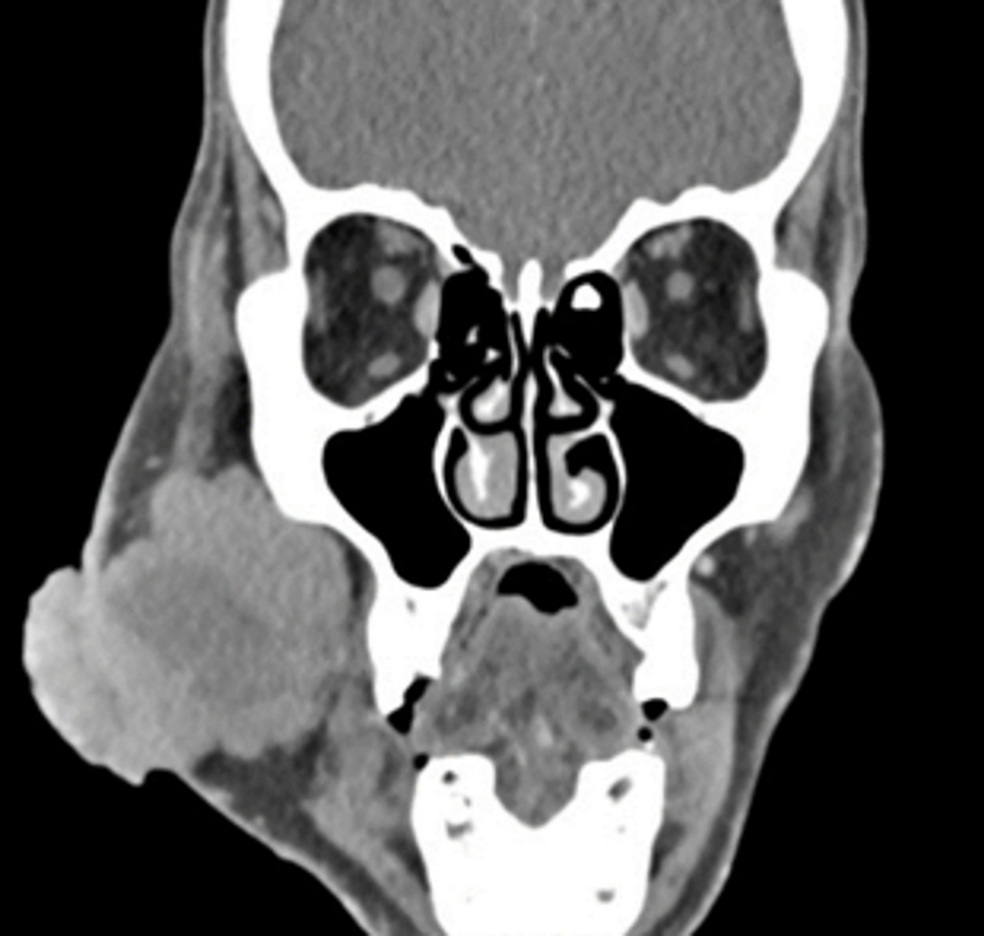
CT scan of the Merkel cell carcinoma, coronal view
